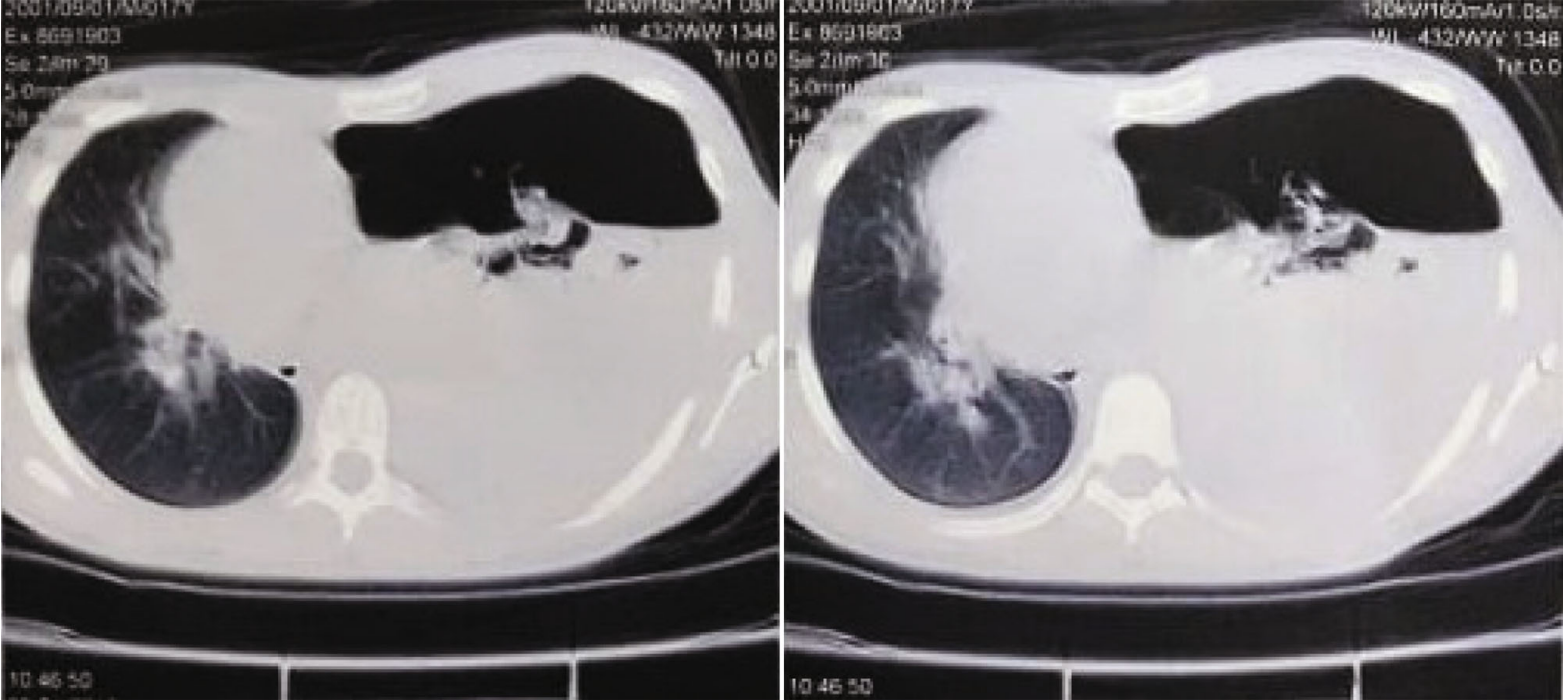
Noncontrast chest CT scan showed hydropneumothorax with left lung collapse and mediastinum deviation.
